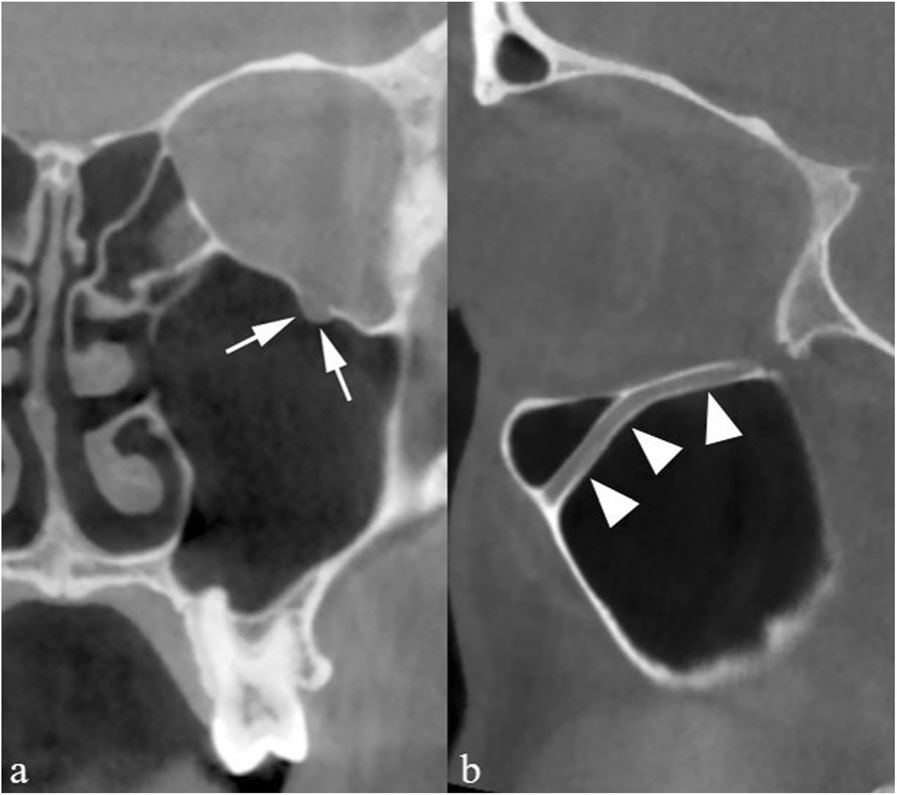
Anatomical variant of nerve course. Coronal CBCT reformation (a) showing dehiscence of the bony walls of the infraorbital canal (arrows) and minor protrusion of the nerve within the maxillary sinus; sagittal CBCT reformation (b) showing the nerve hanging in the maxillary sinus cavity (arrowheads), covered by intact nerve canal walls
